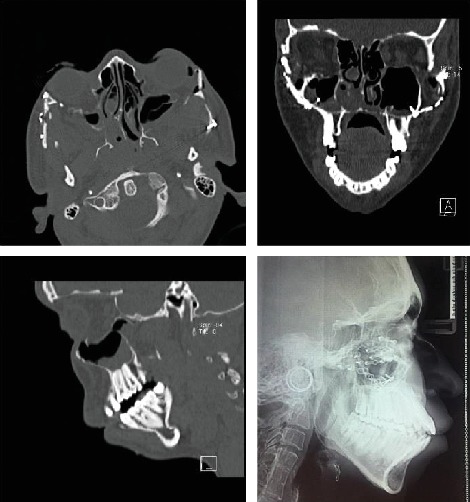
Postreatment axial, sagittal, and coronal computed tomographic views and lateral cephalogram demonstrated correction of orbital depth and exophthalmos.
